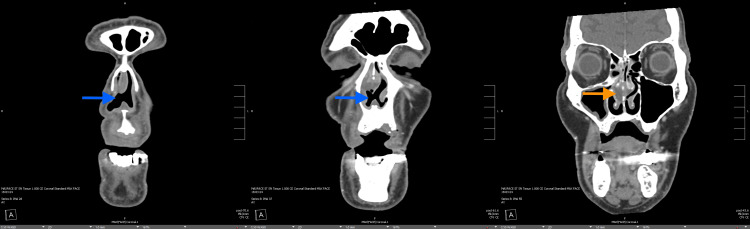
CT maxillofacial without contrast (coronal) concerning for a large nasal septal perforation (blue arrows) and nasal septal abscess (orange arrow) as described in Figure 2
